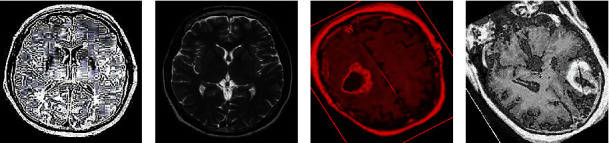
BR35H MRI image: (a, b) nontumorous brain and (c, d) tumorous brain.
